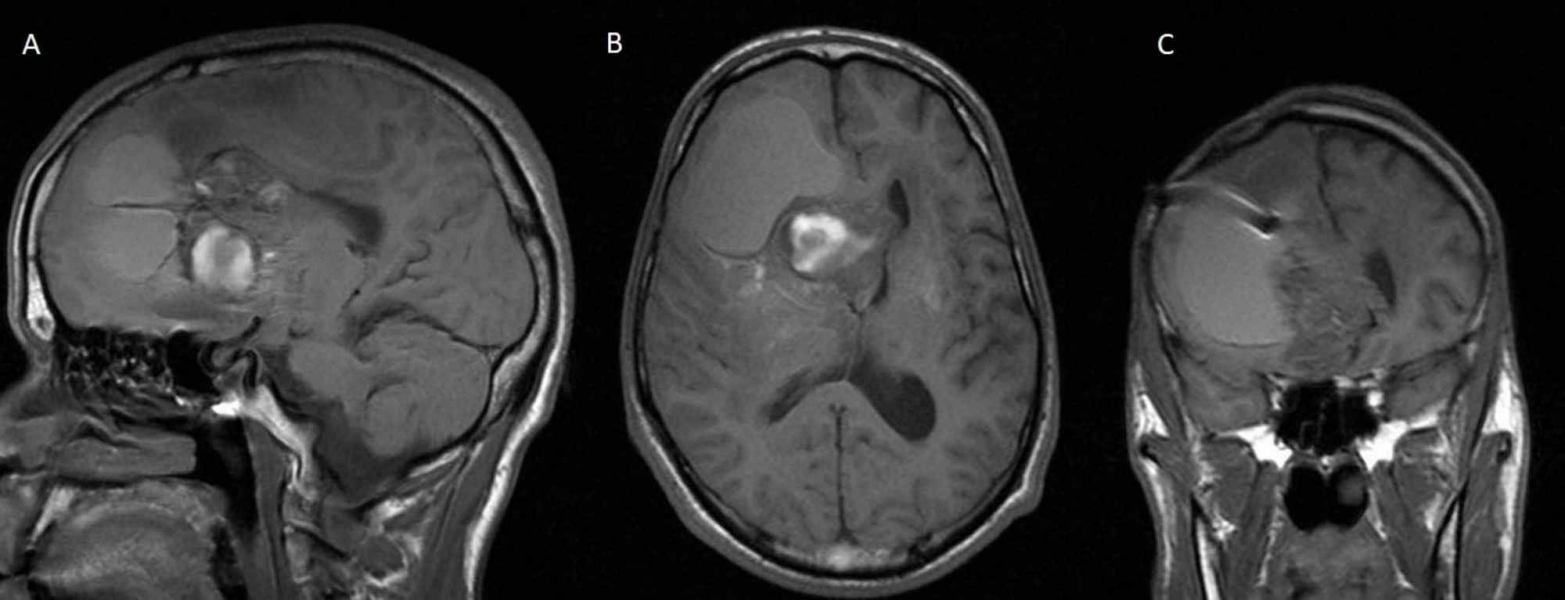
Preoperative sagittal (A), axial (B), and coronal (C) T1-weighted MRI without contrast, showing the solid cystic mass centered near the septum pellucidum. The shunt catheter is seen ending inside the solid mass on the coronal image (c).
